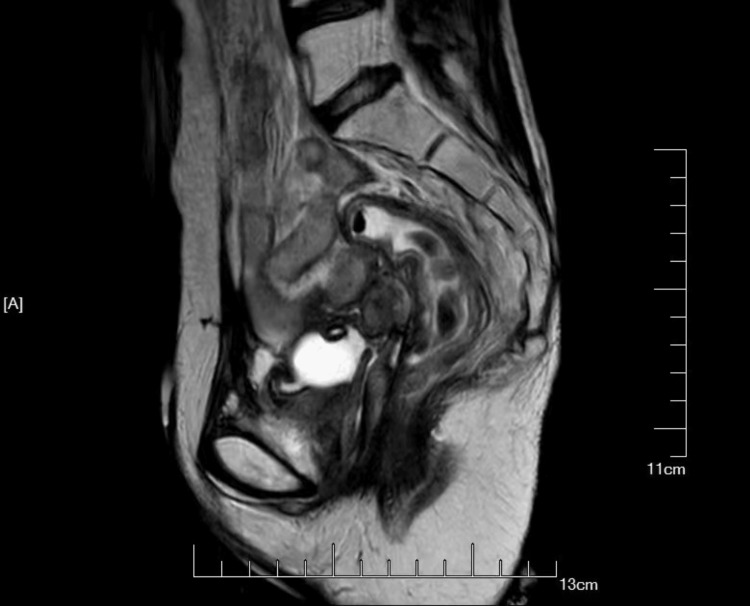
The MRI imaging revealed bladder wall thickening, which may extend to the left ureter. The enhancement of the vaginal stump appeared irregular, with indistinct separation from the bladder, suggesting the possibility of urine fluid leakage.
